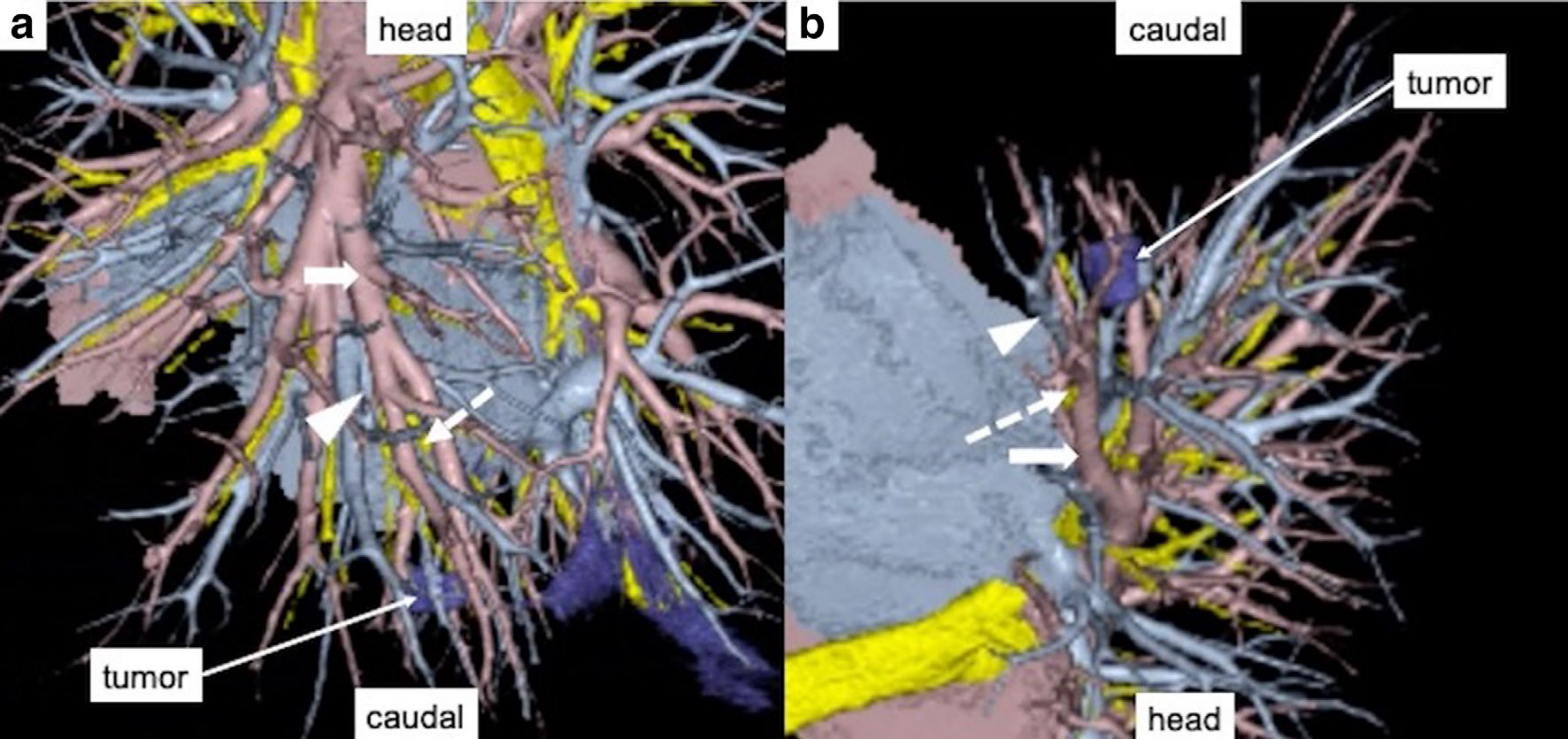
(a, b). Preoperative three‐dimensional CT broncho‐angiography revealed the relative locations of the pulmonary vessels and bronchi. Arrow: Posterior basal branch of the pulmonary artery; dotted arrow: Posterior basal bronchus; arrowhead: Posterior basal branch of the pulmonary vein
